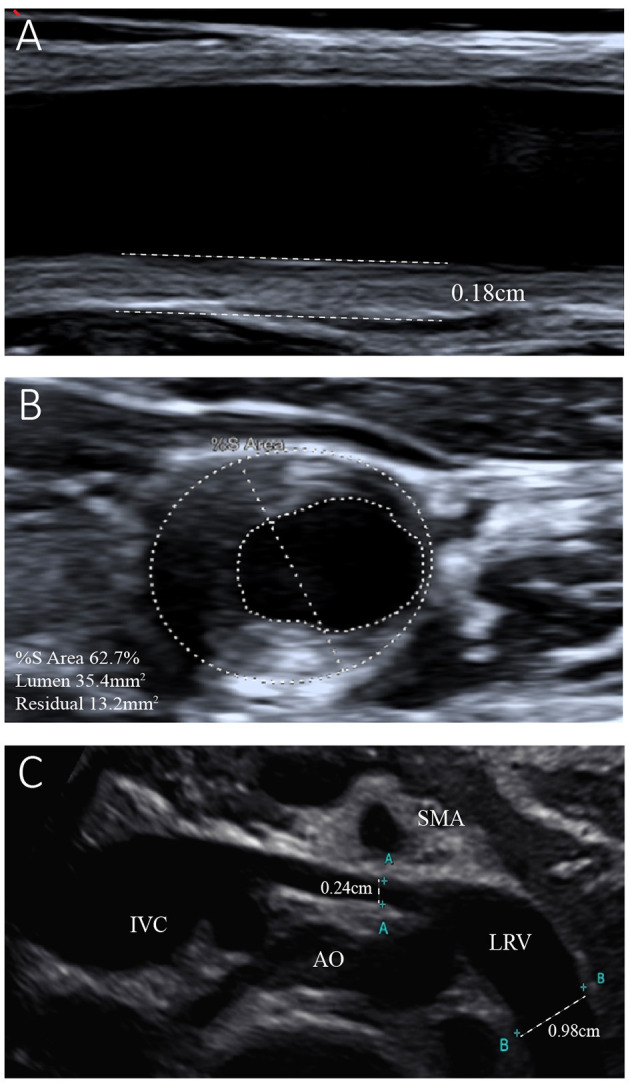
Ultrasonogram of the left common carotid artery and left renal vein. (A) Reveals left carotid atherosclerosis with plaque formation and intima-media thickness of the left common carotid artery of 0.18 cm. (B) Shows 62.7% stenosis of the left common carotid artery. (C) Shows entrapment of the left renal vein (LRV) between the aorta (AO) and superior mesenteric artery (SMA). The internal diameter of the LRV was 0.24 cm, while that of the distal segment was 0.98 cm.
